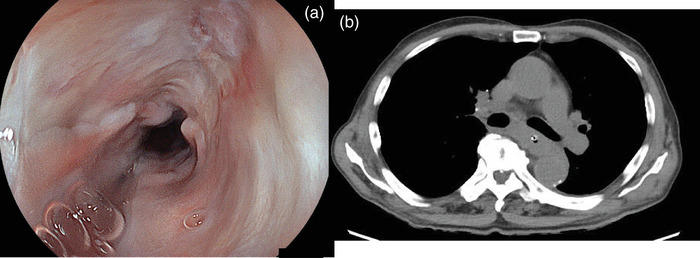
By postoperative day 60, esophagogastroduodenoscopy confirmed that the wound site had completely closed (a). The Computed tomography scan performed on postoperative day 57 showed that the pleural effusion and mediastinal emphysema had resolved (b).
